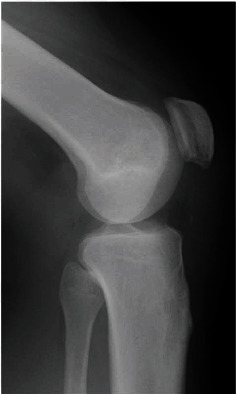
Postoperative lateral radiograph of the left knee at follow-up 12 months after the operation.
